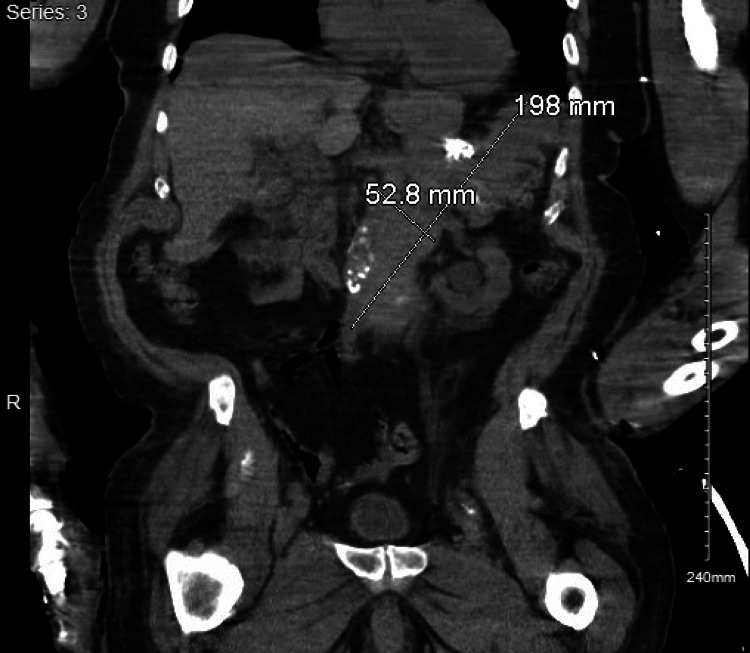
CT finding of a retroperitoneal and left upper quadrant mass with lymphadenopathy CT: Computerized tomography
